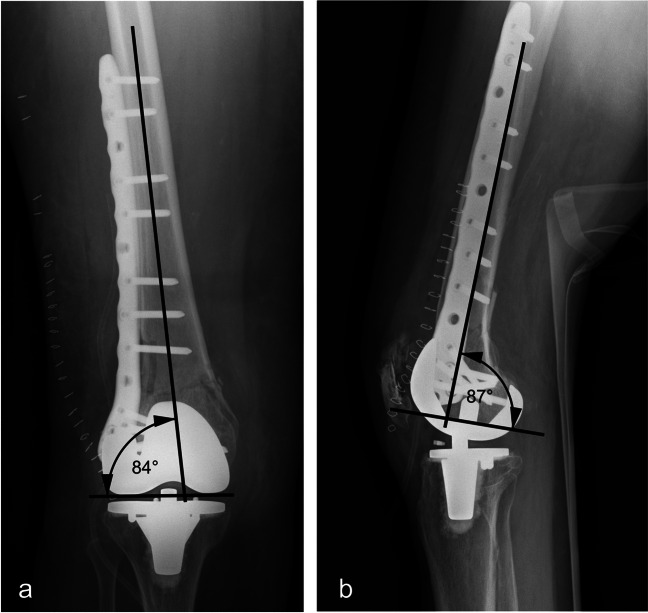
A.p. (a) and lateral (b) view radiographs showing the measuring methods for the aLDFA and aPDFA assessment
